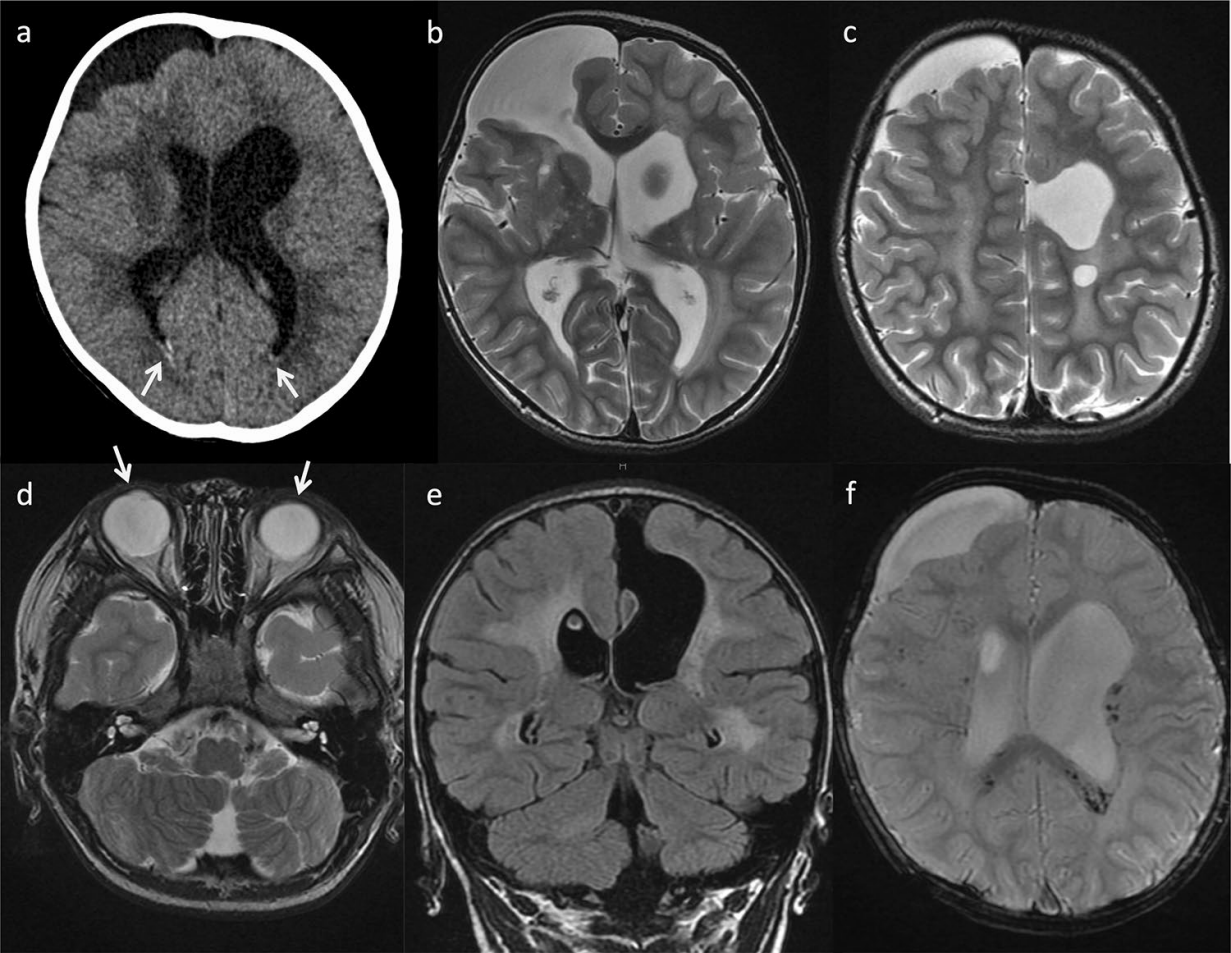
A 3-year-old, male patient with *COL4A1*-related disorder. a Axial non-contrast computed tomography demonstrated calcification along the bilateral occipital horns of the lateral ventricles (arrows) and asymmetrical ventricular enlargement. b-e Axial T2-weighted imaging and coronal FLAIR imaging revealed porencephaly in the bilateral frontal lobes, asymmetrical ventricular enlargement, hyperintensities in the bilateral basal ganglia, thalami, deep white matter, and cerebellar dysplasia. Note also the implanted intraocular lens for cataracts (arrows). f Axial T2*-weighted imaging demonstrated multiple microbleeds in the splenium of the corpus callosum and subcortical and deep white matter
